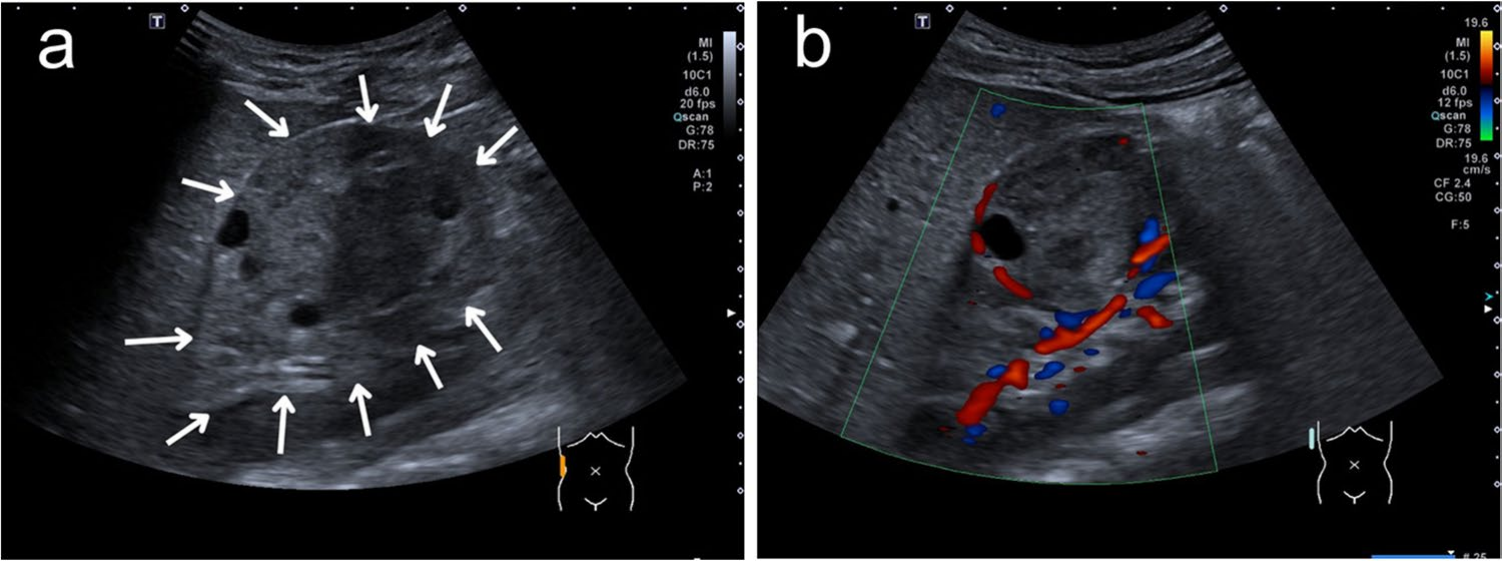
Longitudinal ultrasound image of the right kidney of a 5-year-old boy with a metanephric stromal tumor sharply demarcated from the renal parenchyma (*arrows* in a) and evidence of multiple internal cysts. Significantly reduced perfusion in the color Doppler image (b)
